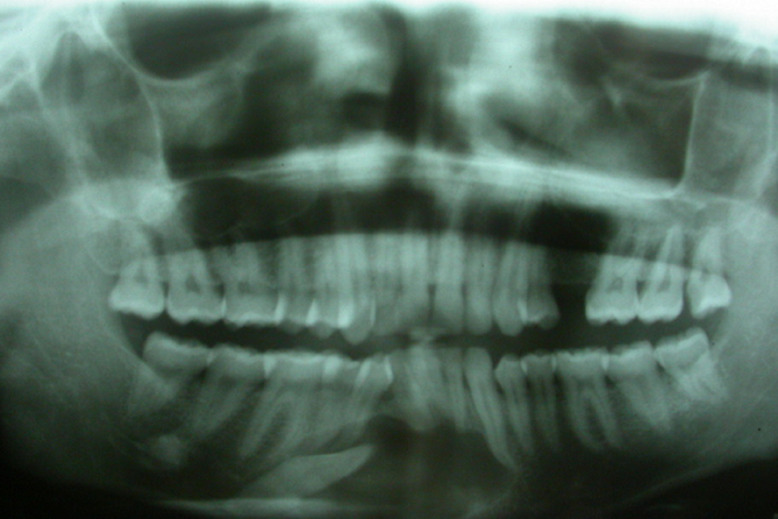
preoperative orthopantomography showing an oval radiolucent lesion in mandible
